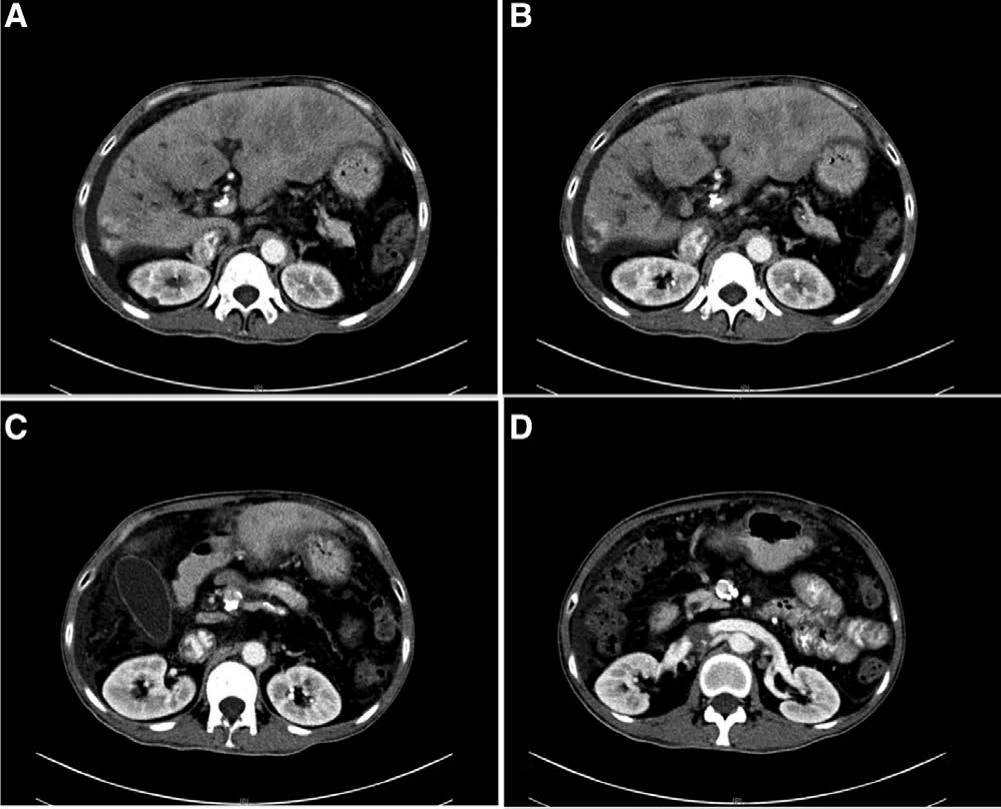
The contrast-enhanced CT of the abdomen of patient. (A and B) Enhanced CT of the abdomen suggests multiple dilation of the intrahepatic bile ducts because of Caroli disease, and liver cirrhosis and portal hypertension. (C and D) Multiple calcifications in the running area of the portal vein and superior mesenteric vein. CT = computed tomography.
